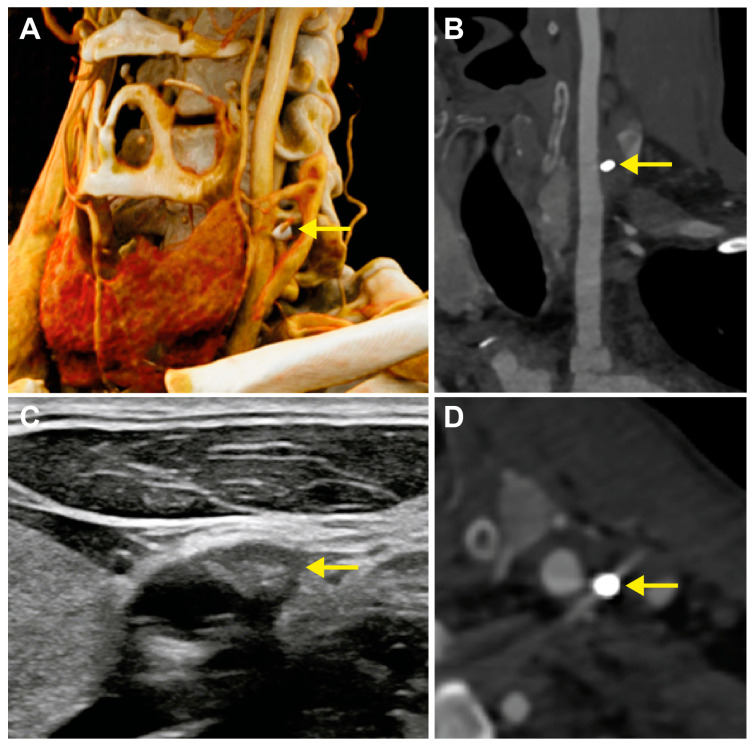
Imaging follow-up. (A,B,D) Computed tomography angiography at 12-months demonstrating the metallic anchor of the MANTA 18-F closure device adjacent to the left common carotid artery (arrows). The carotid artery demonstrates complete patency without stenosis or pseudoaneurysm formation. (C) Duplex ultrasonography on postoperative day 3 showing the extravascular collagen plug (arrow) with normal carotid artery flow.
